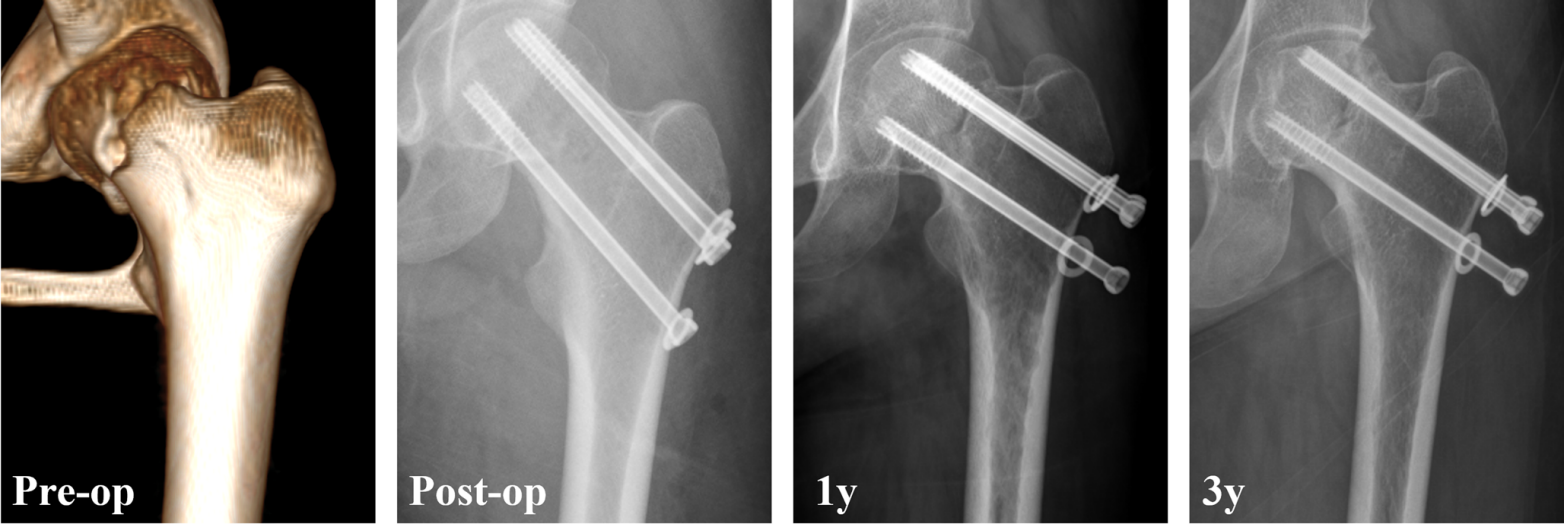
A 32-year-old female who sustained a Pauwels III femoral neck fracture was treated with three cannulated screws. The radiograph taken 1 year postoperatively revealed fixation failure, including femoral neck shortening and non-union. Follow-up at 3 years demonstrated the development of avascular necrosis
